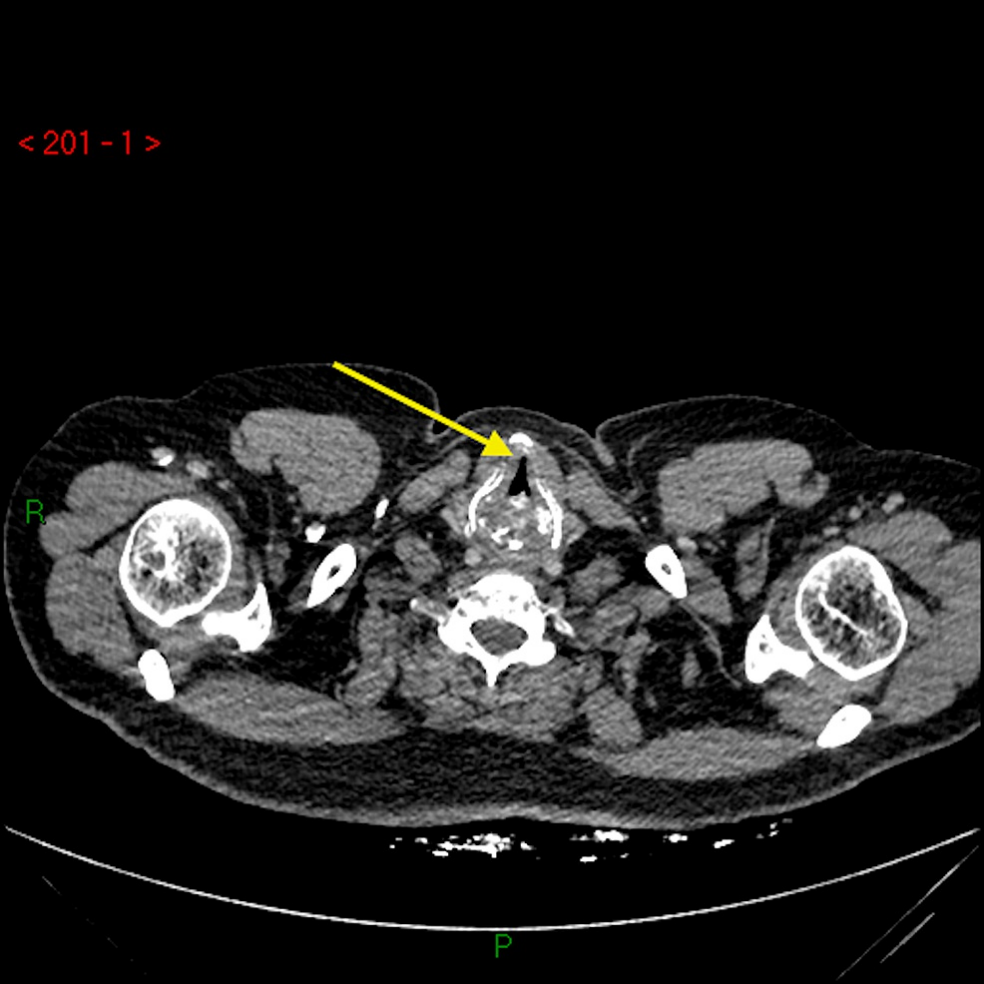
CT demonstrating partially obstructed airway. CT: computed tomography.
